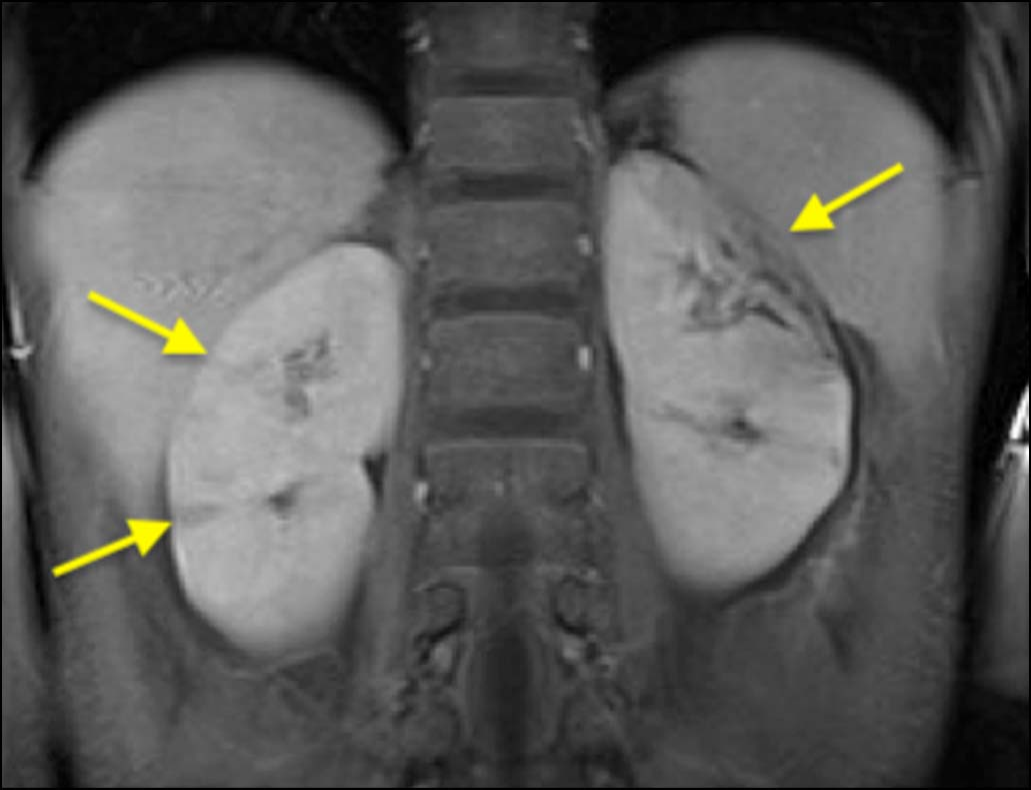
Coronal view of abdominal MRI with abnormal enhancement of the renal parenchyma bilaterally (arrows), with focal atrophic changes on the left.
